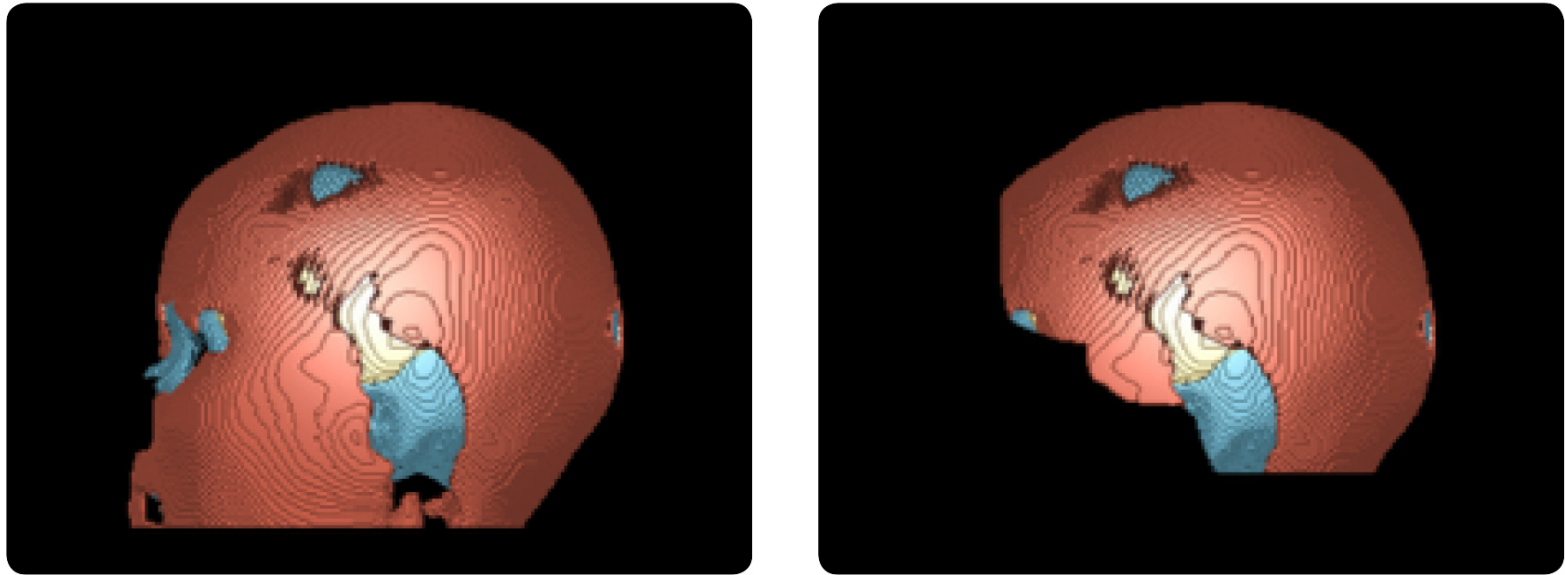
Example of brain ROI cropping. The left panel shows a sagittal view of an original MRI scan and predictions, while the right panel displays the defaced counterparts.
